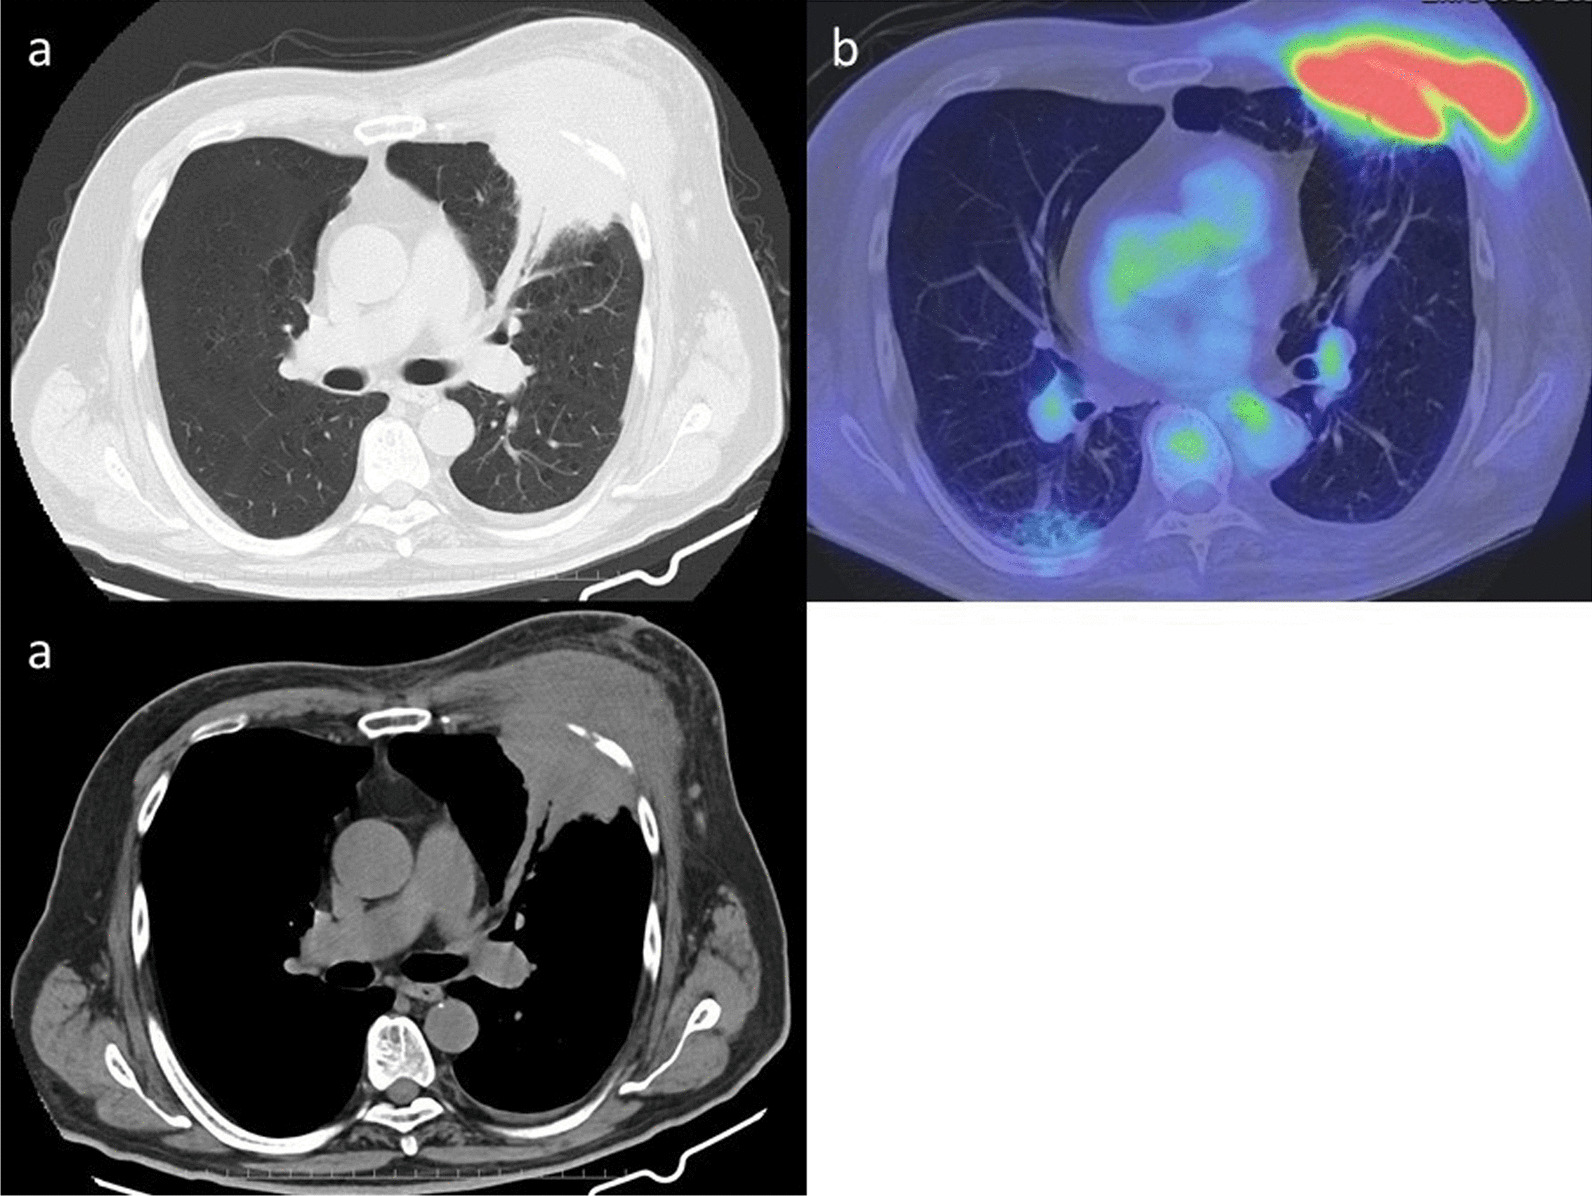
Computed tomography scan image of the chest (a) and ^18^F-fluorodeoxyglucose positron emission tomography image (b) upon presentation
